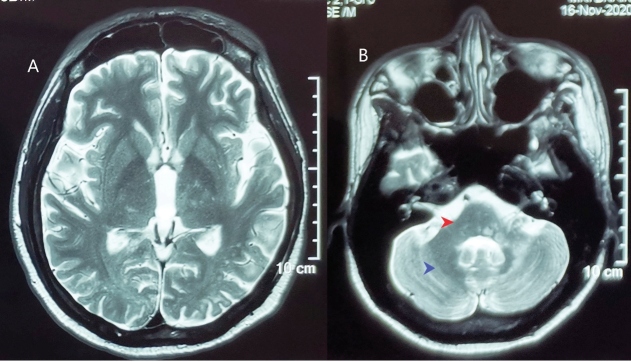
Brain magnetic resonance imaging shows multiple hypointense lesions in (A) the bilateral frontal, parietal, and occipital lobes and (B) the right cerebellar peduncle (red arrow) and cerebellar hemisphere (blue arrow).
